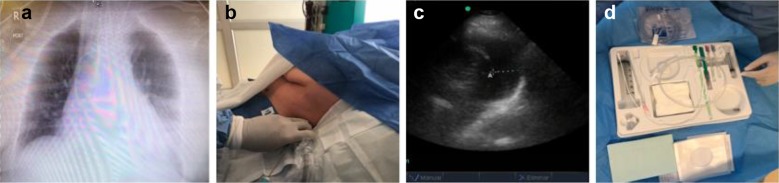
a Chest X-ray of the patient with left pleural effusion during the postoperative period of myocardial revascularization. b Patient in the supine position with the head at 30°; ultrasound assessment in the lower thorax on the posterior axillary line. c Quantification of the pleural effusion with a 5-MHz convex transducer; measurement of the distance between the visceral pleura and the posterior wall of the thorax in the transverse axis. In this case, the distance is 26 mm, with an approximate calculated volume of 520 ml by the Balik method. d Thoracentesis kit
